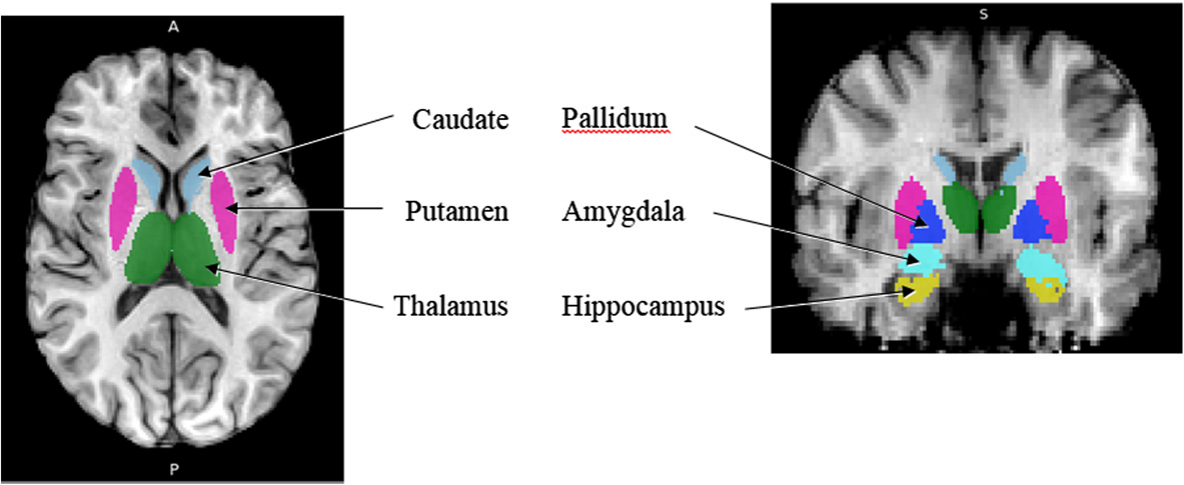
An axial and coronal MRI slice demonstrating the FIRST segmentation of the subcortical deep GM structures.
